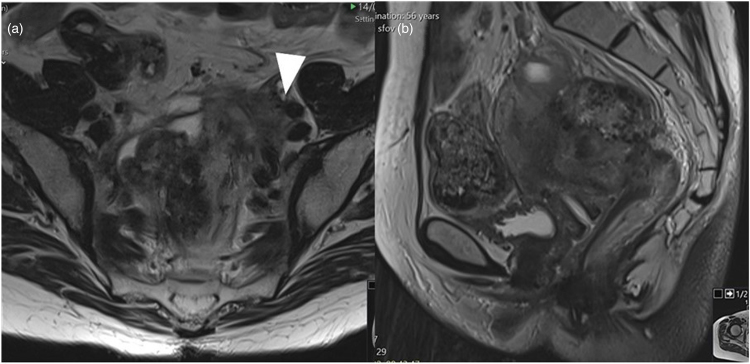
Axial (a) and sagittal (b) pelvic magnetic resonance imaging showing locally recurrent rectal tumour with disease inseparable from the external iliac vessels (arrow head).
